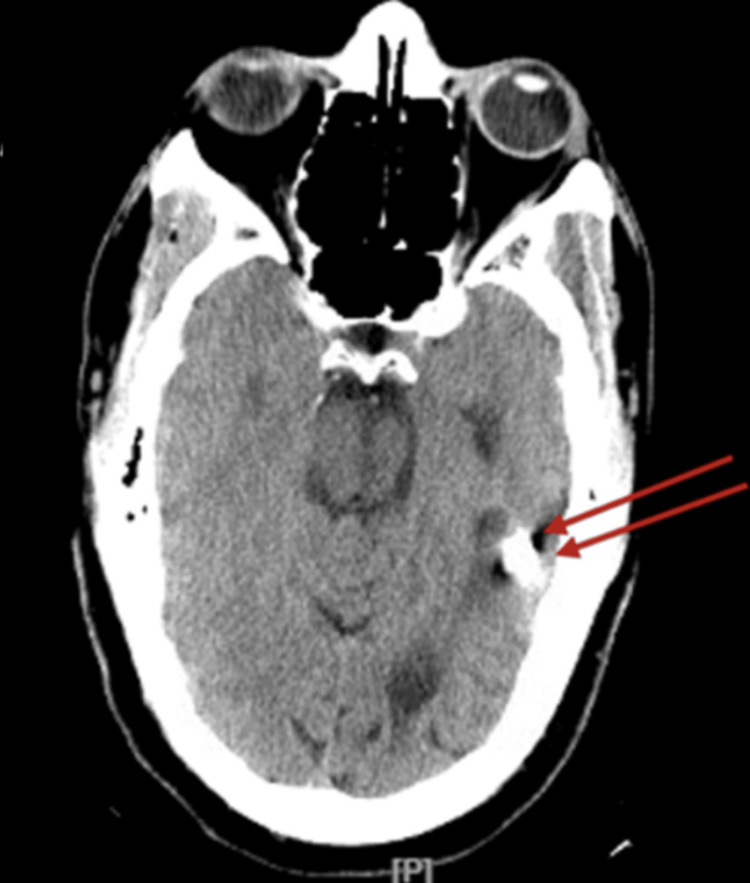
Head CT scan, without contrast. A metallic bullet fragment is identified in the left temporoparietal region from a prior gunshot wound. Basal ganglia old infarct with gliosis.
